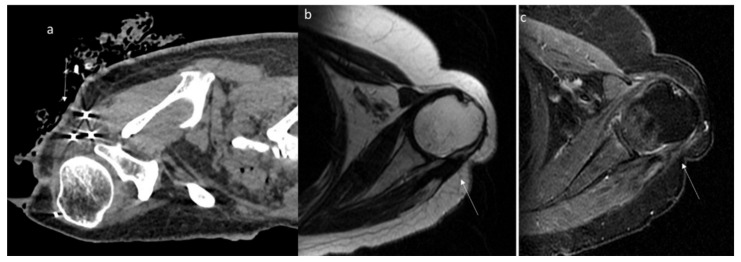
The same patient of Figure 4, treated by CT-guided cryoablation (probes and ice-ball within the lesion in (a); the follow-up control after 6 months depicts evident volumetric and enhancement reduction (arrows) of the lesion (b,c).
